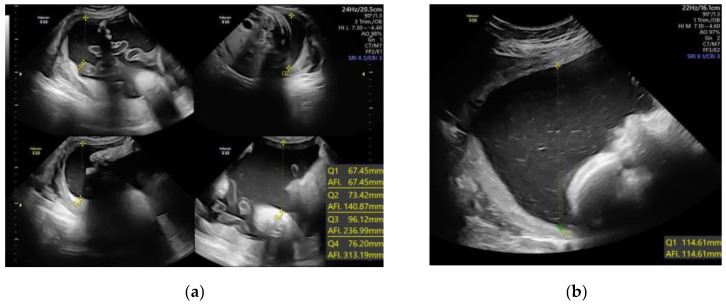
Amniotic fluid volume determination using quantitative sonographic techniques: (a) Amniotic fluid index (AFI); (b) single deepest pocket (SDP).
